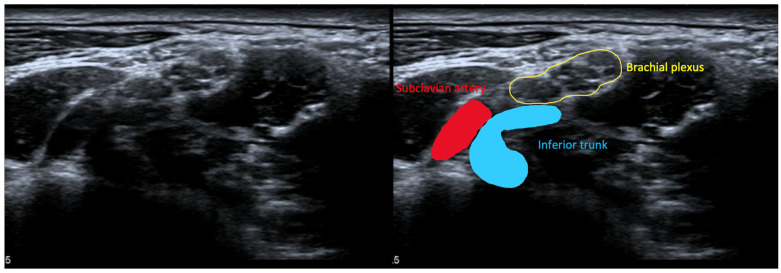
A wedge-sickle sign (blue) seen in high-resolution ultrasound pictures in patients with GSH.
